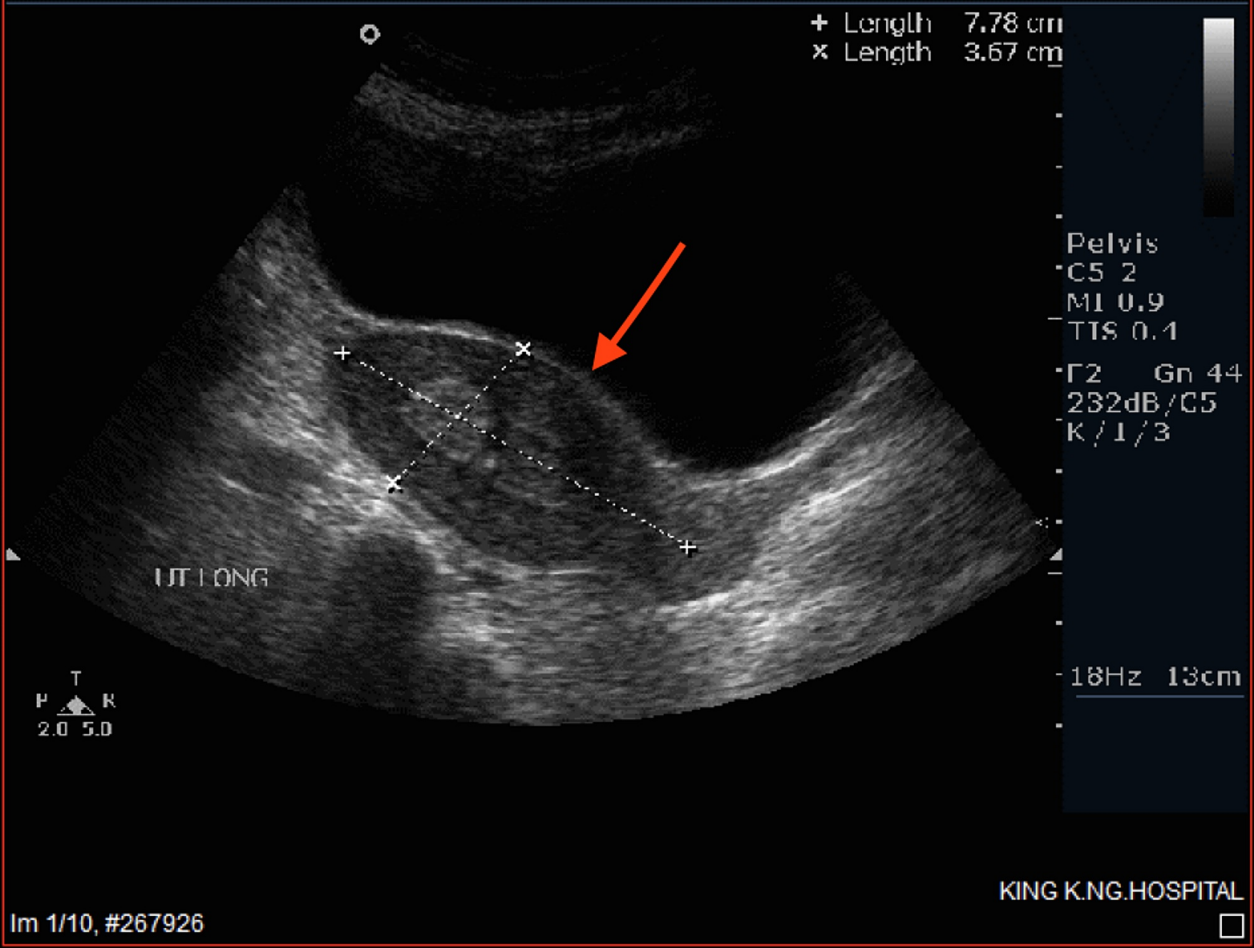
Ultrasound showing endometrial polyp measuring 1.5x2 cm
